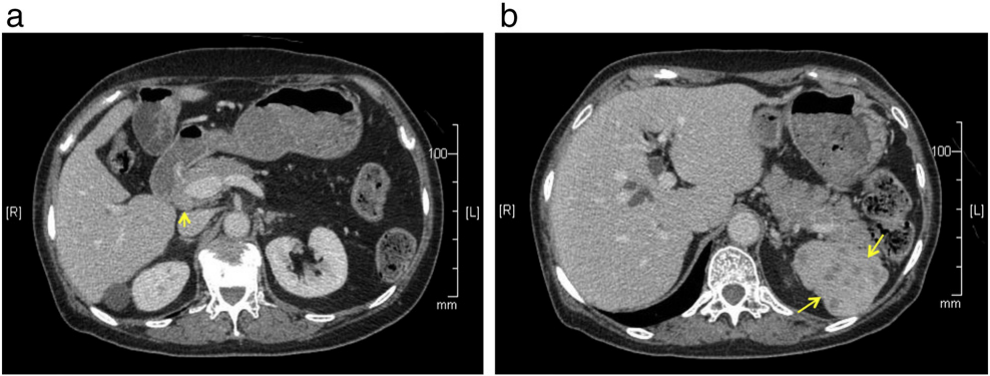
(a) CT scan showing swellings in the lymph nodes (arrows). (b) Low-density areas in the spleen were also observed (arrows), suggesting metastasis from a malignant tumor of the left ovary.
